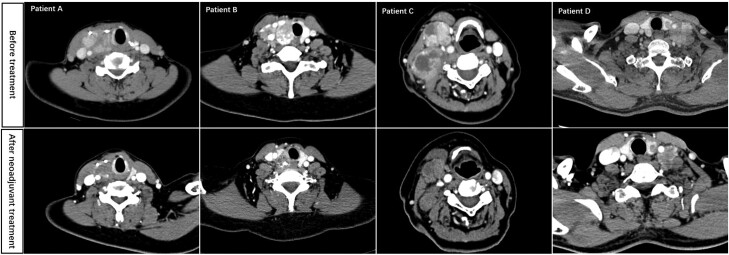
The computed tomography scans showing tumor changes before and after neoadjuvant treatment. Patient A: Thyroid tumor decreased in size by 37.3%. Patient B: The target lesions decreased 36.3%. The primary lesion developed liquefaction necrosis. The right internal jugular vein was no longer compressed by lymph nodes after 2 cycles of apatinib treatment. Patient C: The lymph node at the right neck decreased in size by 33.7%. Patient D: The metastatic lymph node at the left neck decreased and the left common carotid artery was no longer surrounded by lymph nodes.
